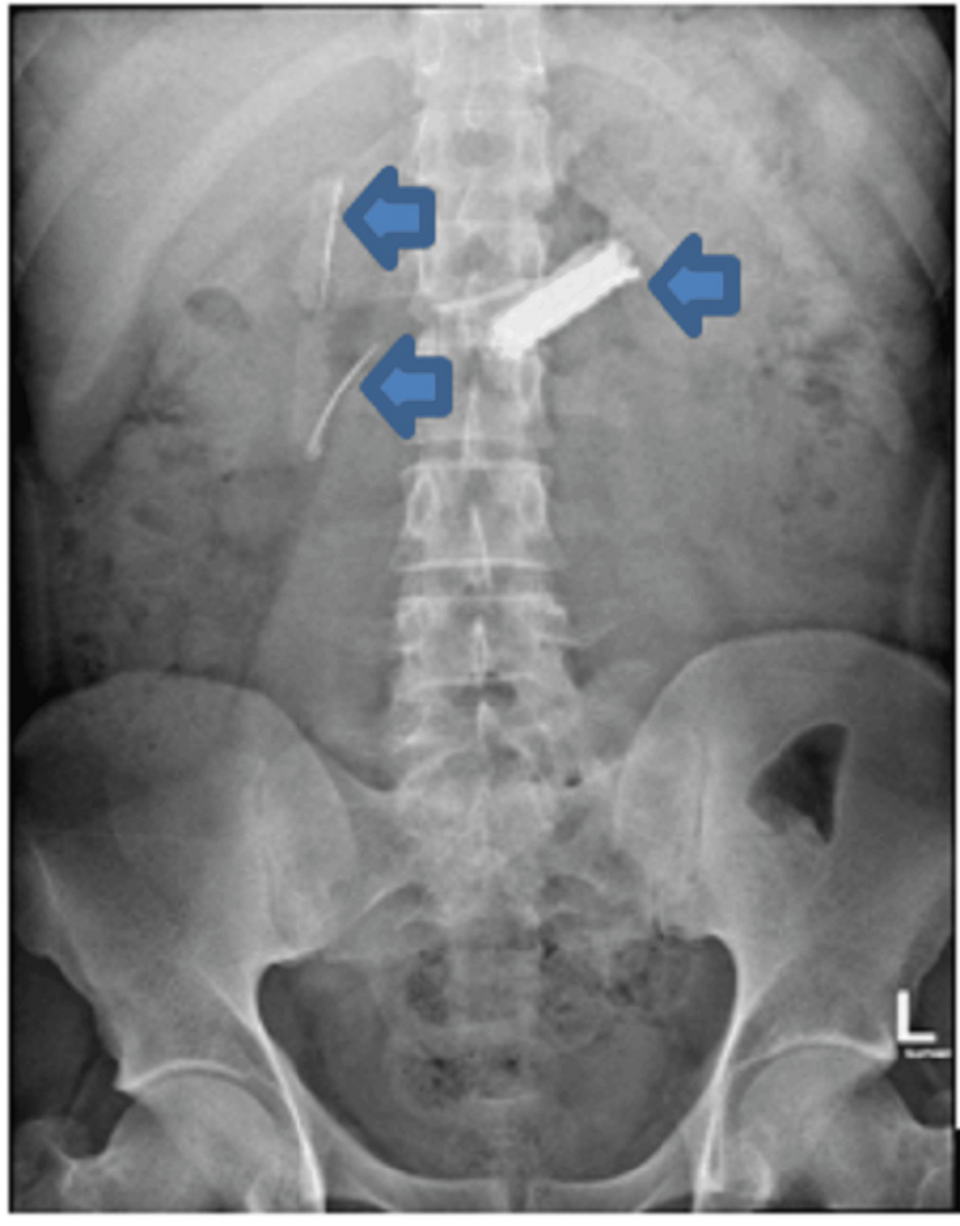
X-rays of the abdomen show sharp objects located in the stomach
